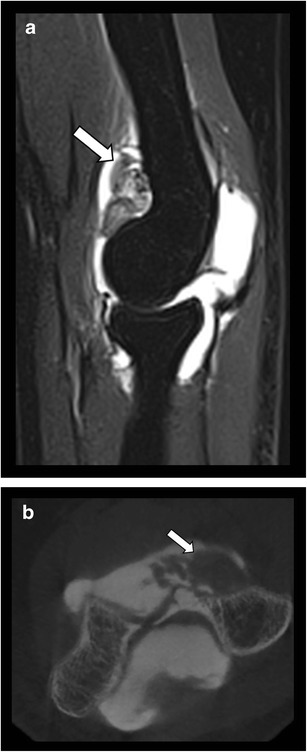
Pigmented villonodular synovitis of the elbow. a. Sagittal T2–WI fat suppressed MRI image presenting a mass within the joint cavity (arrow) with a low signal areas related to hemosiderin deposits. b. CBCT-A showing the presence of proliferative synovium (arrow) within the anterior part of the joint cavity
